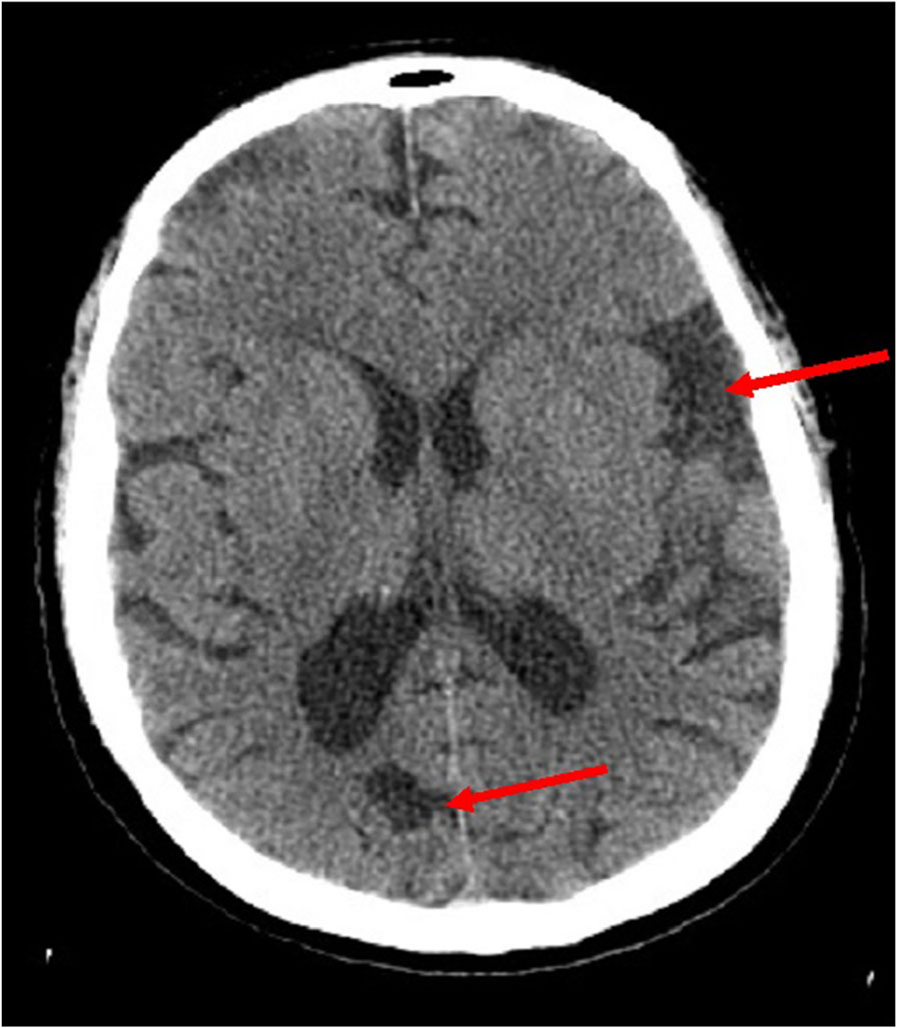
Computed tomography scan of brain without contrast showing brain atrophy disproportionately advanced for patient’s age. *Arrows* pointing to areas of atrophy
